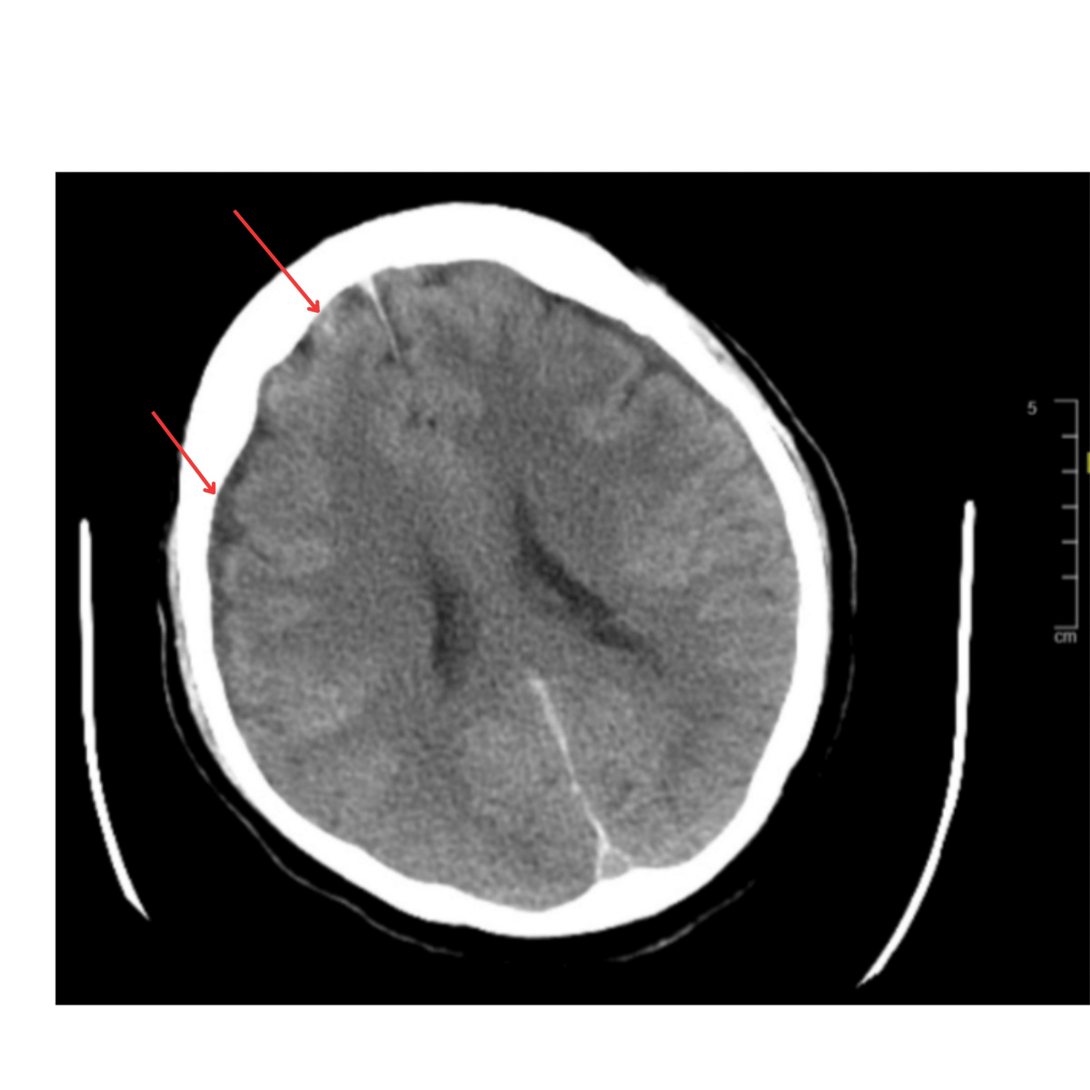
CT head without contrast CT: Computed tomography The top left arrow is pointing to the minor right frontal subarachnoid hemorrhage. The bottom left arrow is pointing to a small hemorrhage in the right frontoparietal sulci
